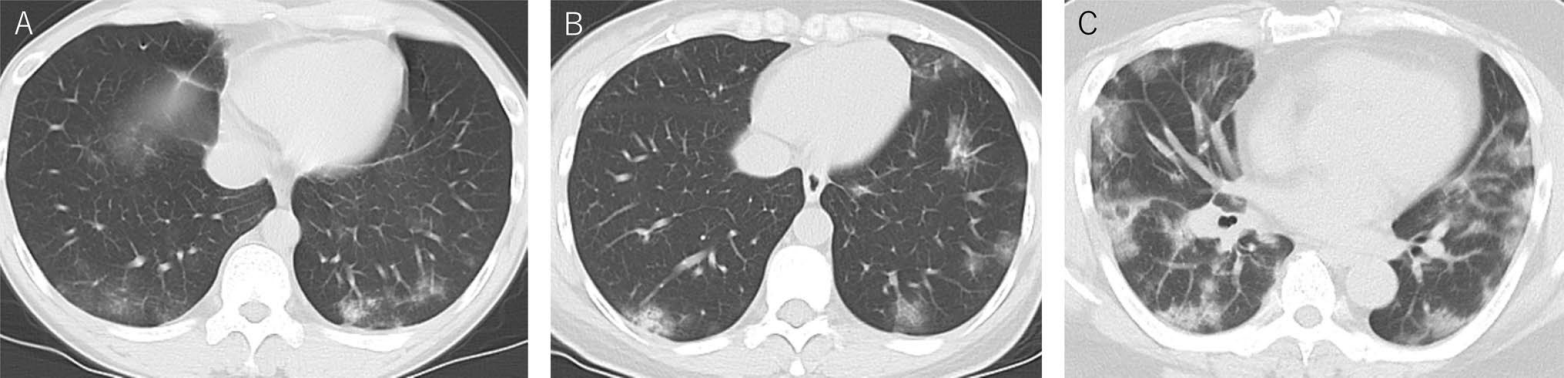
A A 46-year-old woman infected with SARS-CoV-2 wild type. On axial CT image on day 3, non-segmental ground-glass opacities were demonstrated in the left and right lower lobes. B A 39-year-old woman infected with SARS-CoV-2 Alpha variant. On axial CT image on day 3, non-segmental patchy ground-glass opacities were demonstrated in the left lower and upper lobes and right lower and middle lobes. C A 52-year-old man infected with SARS-CoV-2 Delta variant. On axial CT image on day 3, non-segmental ground-glass diffuse opacities were demonstrated in the left lower and upper lobes and right lower and middle lobes
